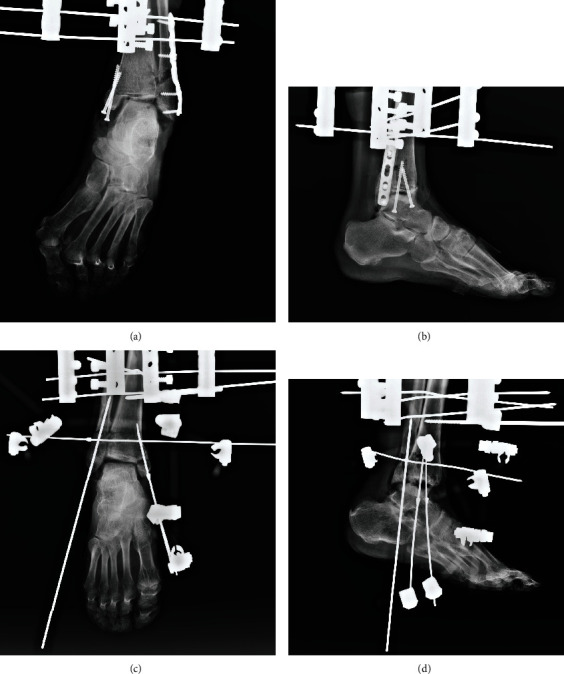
The anteroposterior and lateral radiographs were taken after fixation. (a, b) The internal fixation. (c, d) The nonbridging external fixation.
